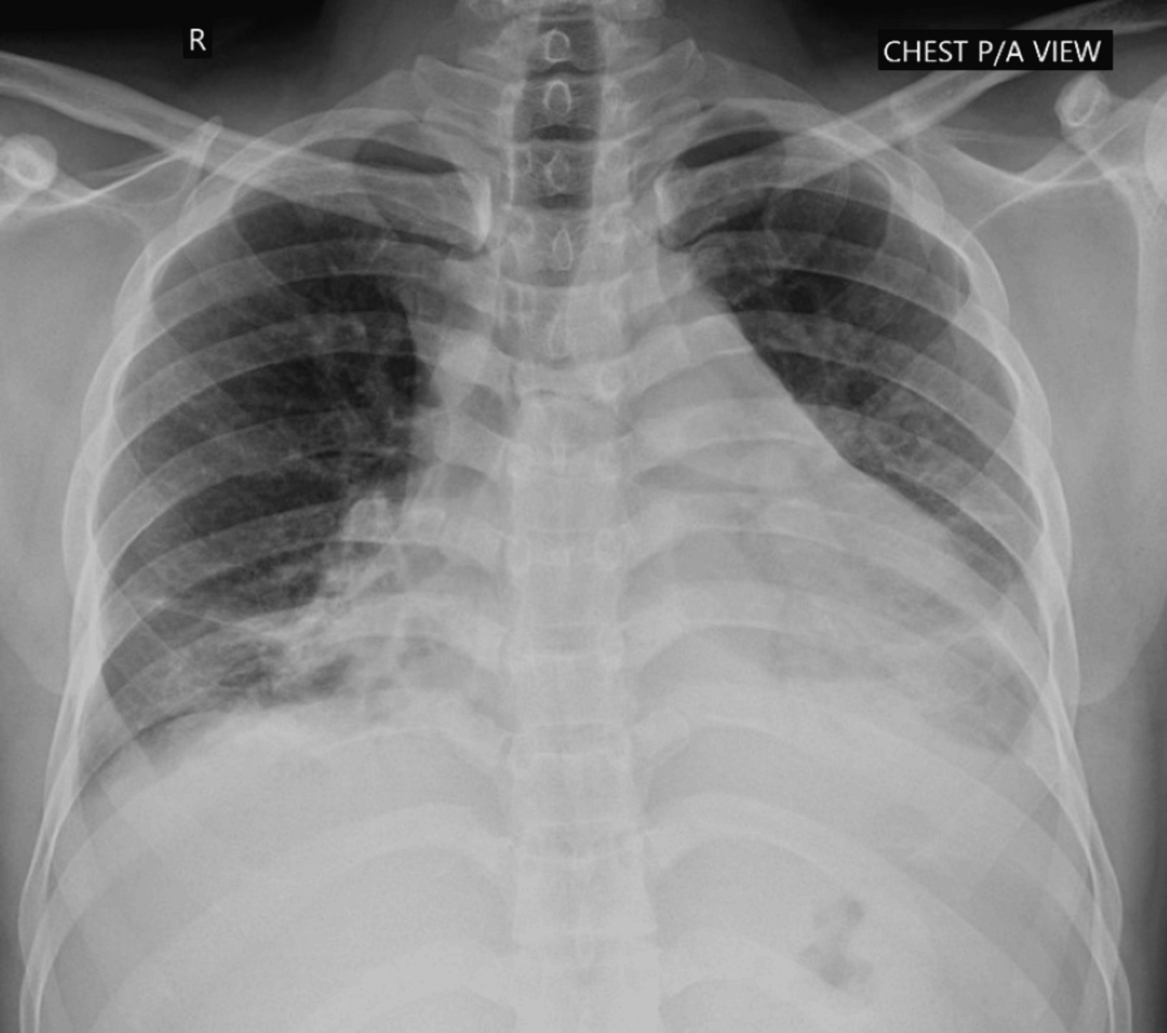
Chest X-ray at the time of admission showed enlargement of the cardiac shadow and a little bilateral pleural effusion.
